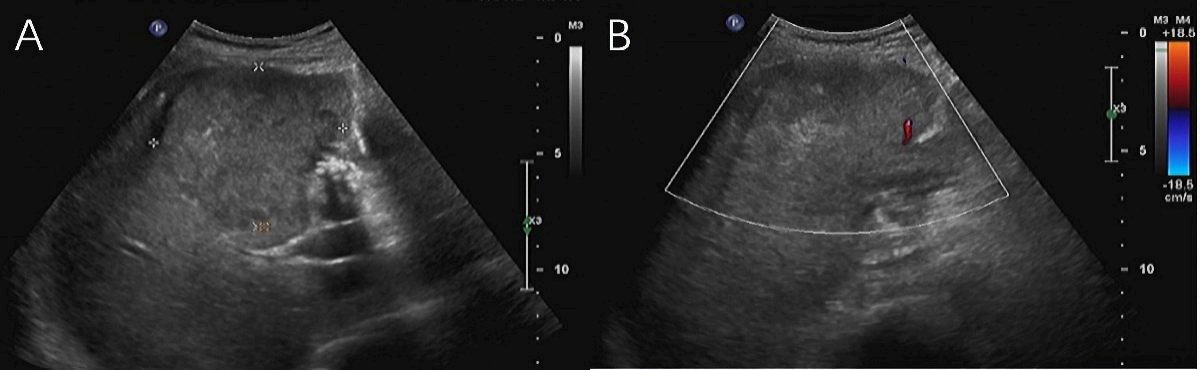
(A) Ultrasound imaging revealed a large, solid, hypoechoic mass with unclear margins and heterogeneous internal echo patterns located in the right anterior lobe of the liver. (B) Color Doppler imaging showed limited blood flow signals within the mass.
